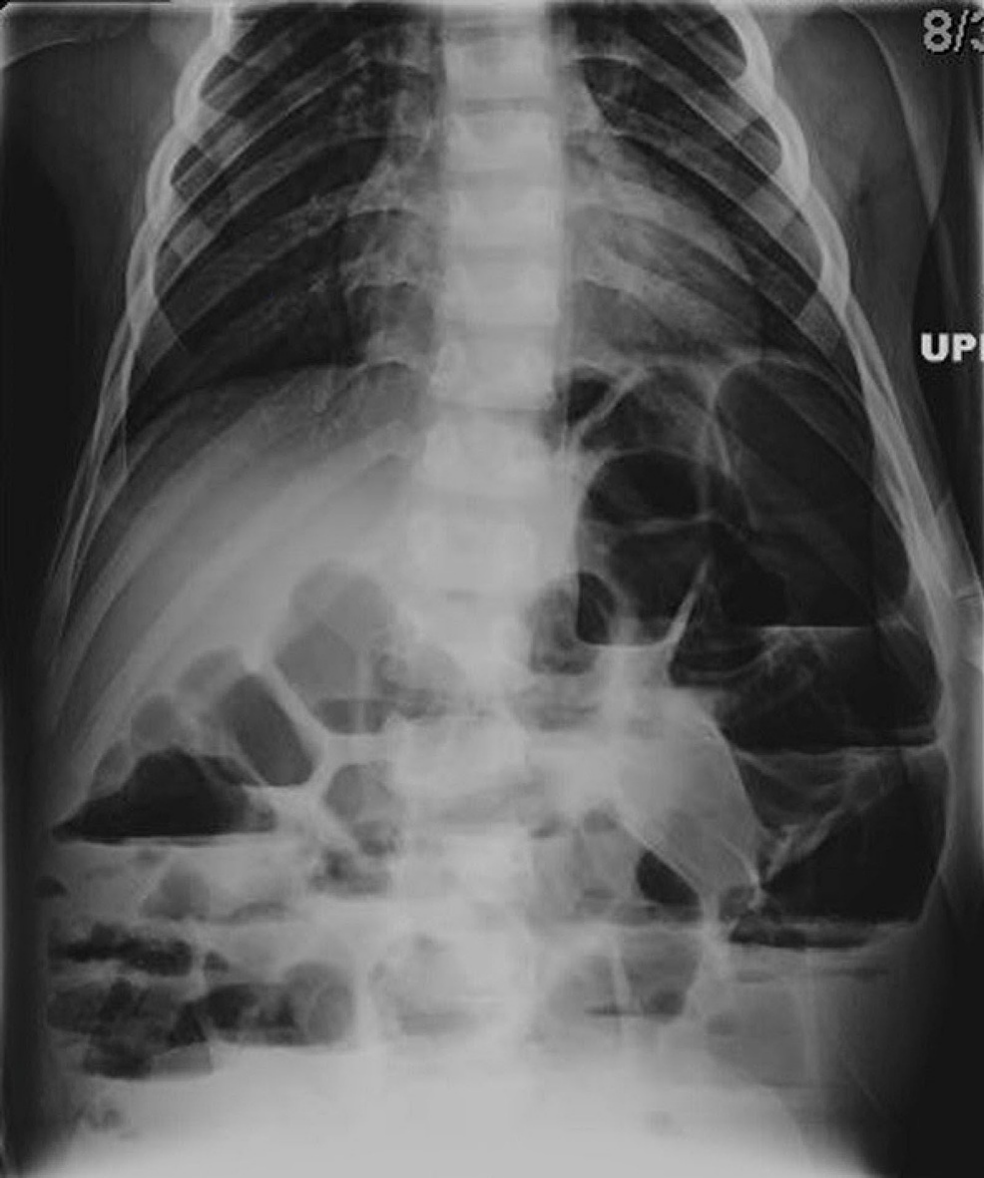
Upright abdominal X-ray showing dilated colon
